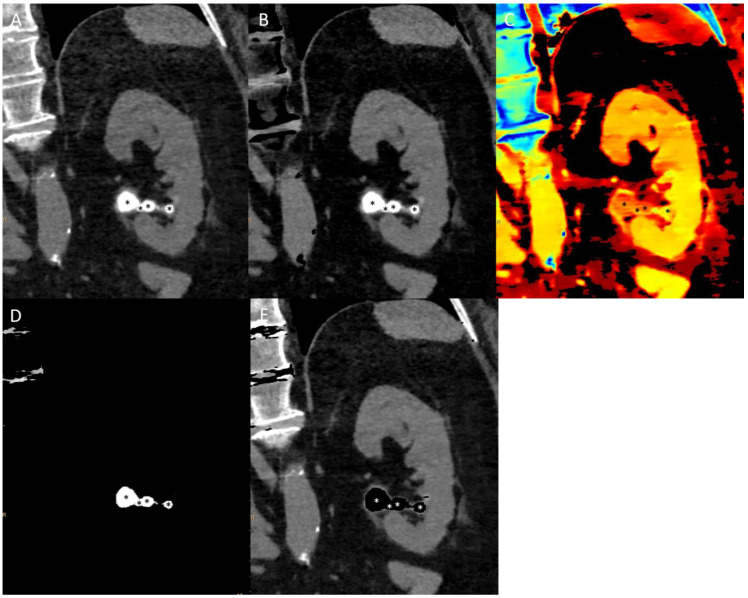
Non-contrast CT scan of uric acid kidney stones (*) in para-coronal reformations: conventional multi-energetic CT image (A), calcium suppression (B), Z-effective maps image (C), uric acid only image (D), and uric acid suppression image (E). Note the absence of suppression in B and the difference in Z-effective images between stones and spine bones, which are rich in calcium.
